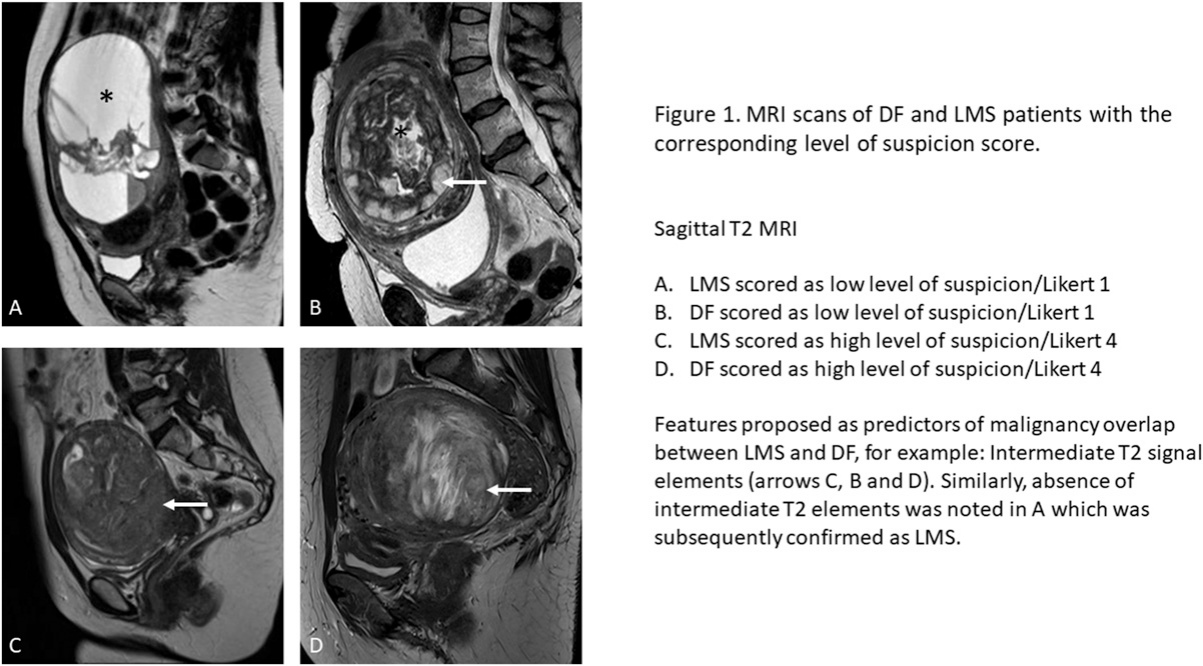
MRI scans of DF and LMS patients with the corresponding level of suspicion score.
